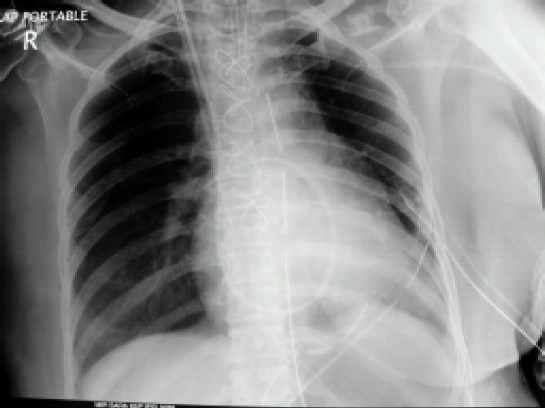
Chest radiograph showing a well-expanded lung after bronchoscopic clot evacuation.
